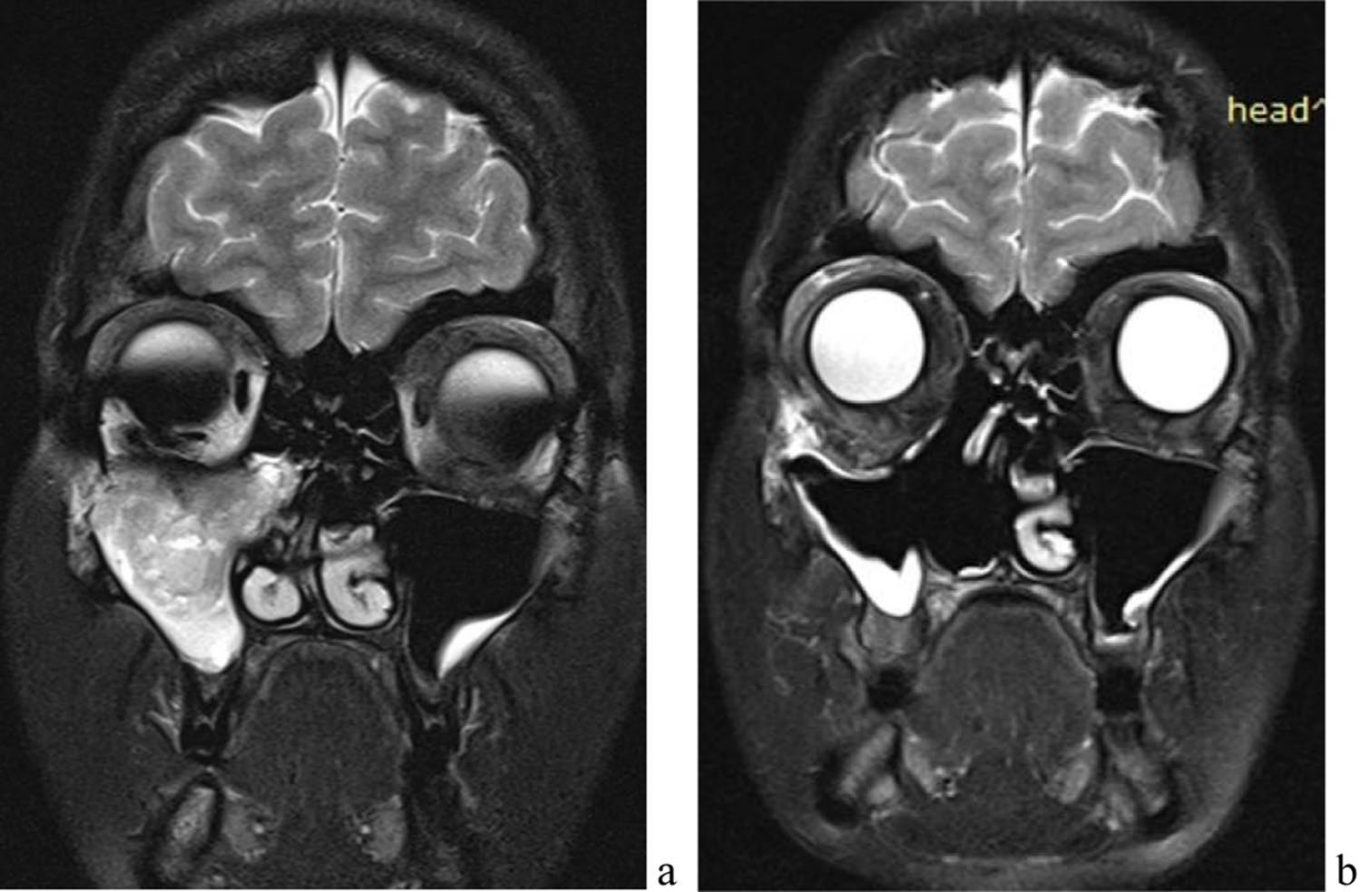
Pre- and postoperative MRI scan (Case 2)
